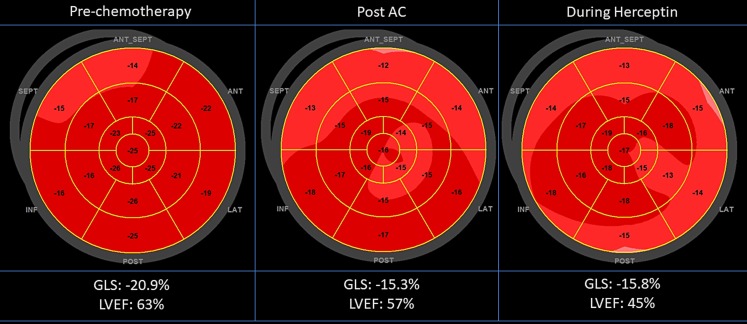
Echocardiographic deformation imaging. Longitudinal follow-up of a 51-year-old female with breast cancer with a high cardiovascular risk (Cardiotoxicity Risk Score 7: female, hypertension, concurrent anthracyclines, and high-risk agent trastuzumab). After the initial 4 × AC (adriamycin-cyclophosphamide) there was a significant decrease of >15% in global longitudinal strain (*GLS*) with preservation of left ventricular ejection fraction (*LVEF*). During the trastuzumab treatment there was a subsequent decrease in LVEF of >10%. After interruption of the trastuzumab treatment, the LVEF showed a complete recovery
